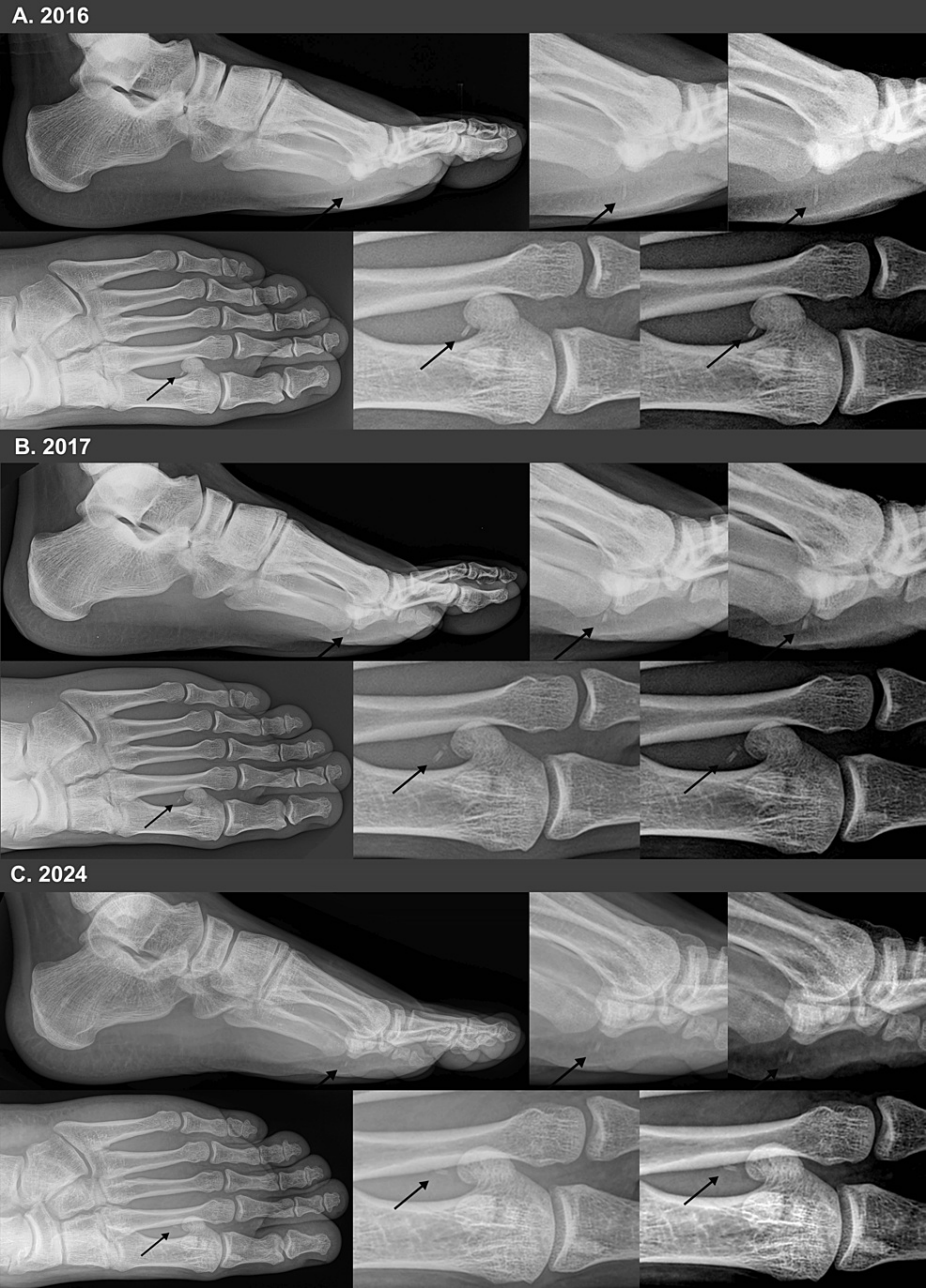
Lateral and anterior-posterior (or oblique) radiographs of the patient’s left foot A: three weeks after the injury; B: one year after the injury; and C: eight years after the injury. Arrows point to the embedded spine. In B, the spine was noted to be fragmented. Radiographs on the far right of each row have enhanced contrast in order to better visualize the sea urchin spine; all other radiographs are as seen in the clinic. The embedded spine resembles that shown in a radiograph of a 31-year-old female, where the spine was embedded in the posterior tibia tendon (and was surgically removed soon after the injury) [10].
